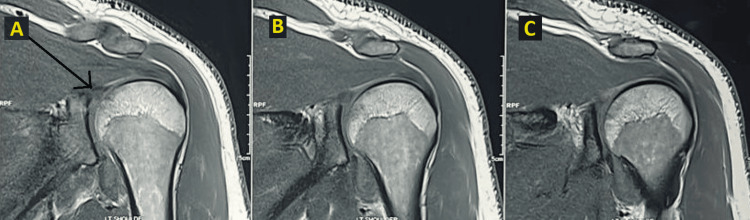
MRI of the left shoulder joint A)-C) Serial coronal MRI cuts of the left shoulder joint The black arrow shows the lesion
